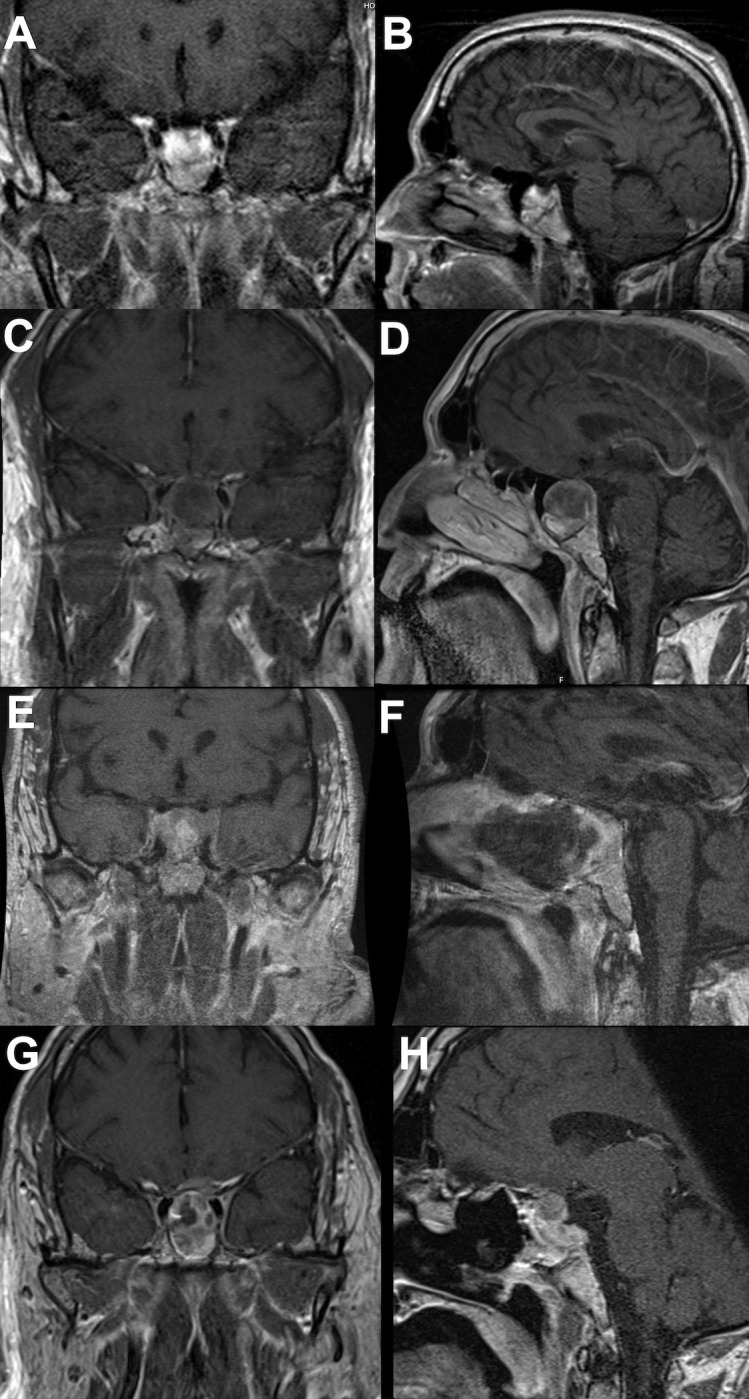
Brain MRI showing tumor progression. (A coronal; B sagittal) showing initial tumor presentation. (C coronal; D sagittal) showing tumor prior to first operation. (E coronal; F sagittal) showing tumor after first operation. (G coronal; H sagittal) showing continued tumor progression postoperatively and prior to temozolomide therapy.
